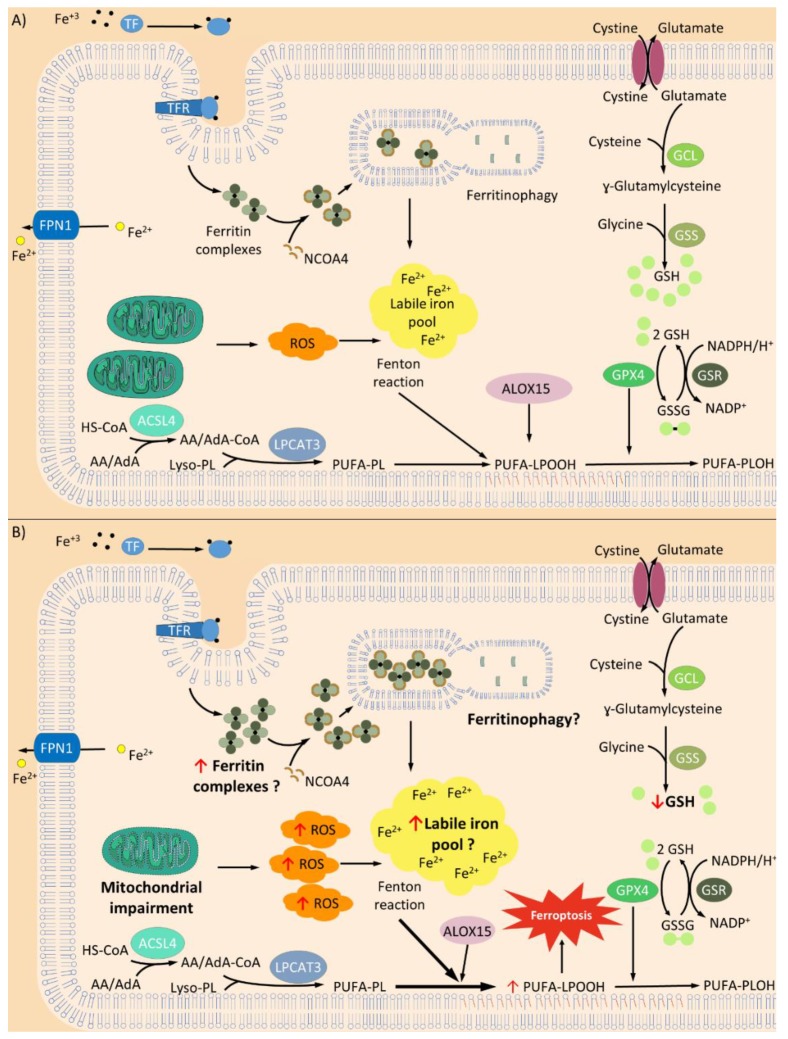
Main mechanisms in ferroptosis. (A) General process of ferroptosis. Balanced levels of reactive oxygen species (ROS) generation and antioxidant activity maintains an inactive ferroptosis cell death pathway; however, some disturbance in the players of this equilibrium could produce a ferroptosis activation. Two important components of ferroptosis are the process of transferrin (TF)-iron import and ferroportin (FPN1) export. Changes in iron uptake or iron export produce a suppression or activation of ferroptosis since the free iron pool is fundamental [31,32]. Ferroptosis may be enhanced through the process of ferritinophagy, by which ferritin is targeted by nuclear receptor coactivator 4 (NCOA4) and delivered to the lysosome for degradation in autophagosome [33,34,35]. Consequently, a large quantity of iron can be rapidly released. Currently, it has been suggested that ferroptosis initiation might be triggered by an increase in free iron levels itself [36]. Iron increase/or accumulation induces the Fenton reaction which increases the production of ROS. Furthermore, the action of iron-dependent oxidases [37], specifically lipoxygenase activity of 15-LOX (ALOX15) that oxidizes polyunsaturated fatty acids (PUFAs) phospholipids (PUFA-PLs) containing arachidonate or adrenate moieties, triggers the ferroptosis pathway [38,39]. In addition, increased activity of acyl-CoA synthetase long-chain family member 4 (ACSL4), an enzyme that preferentially activates arachidonic (AA) and adrenoic acids (AdA), has been reported to increase ferroptosis [38,40]. Inhibition of glutathione peroxidase enzyme 4 (GPX4) [2,3] or glutathione (GSH) unavailability [4,5] produces lipid hydroperoxide accumulation that triggers ferroptosis. (B) Suggested mechanisms of ferroptosis in Friedreich’s ataxia (FRDA). It has been observed increased levels of lipoperoxides and ROS in FRDA neurons. Furthermore, in these neurons, it has also been reported lower reduced GSH concentration and GSH homeostasis disturbance, respectively [41,42,43]. Controversial studies are reporting either normal or increased iron levels in the nervous system of FRDA patients (reviewed in [44]). Recently a study in FRDA patients, using magnetic resonance imaging, showed increased iron concentration in the extrapyramidal motor system compared with controls [45]. However, there is no information about distribution, location and redox state of the iron in cells from FRDA (indicated with “?”). Increased autophagic processes are related to FRDA in different models of disease [46,47], but there is no information about ferritinophagic activity. Further studies should be performed in order to unravel if this mechanism increases the iron content in FRDA cells. All these ferroptotic characteristics observed in FRDA may indicate that this kind of cell death may have a role in the physiopathology of this disease. Transferrin receptor (TFR) Lysophosphatidylcholine Acyltransferase 3 (LPCAT3); Glutamate Cysteine Ligase (GCL); Glutathione synthetase (GSS); Glutathione reductase (GR). ↑ indicates increased levels and ↓ indicates decreased levels.
